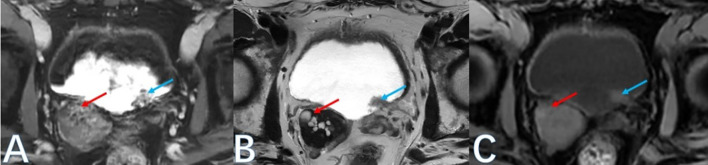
Magnetic resonance imaging (MRI) images of Case 2 demonstrating two distinct synchronous pelvic lesions. (A) Axial T1-weighted image (T1WI) showing a cauliflower-like soft-tissue lesion on the left posterior bladder wall with hypointense signal (blue arrow, ~12 × 8 mm) and a separate heterogeneous mass located in the right pelvis inseparable from the right seminal vesicle with hypointense signal (red arrow, ~3.2 × 3.1 cm). The prostate is enlarged with focal T1-hypointense areas. (B) Axial T2-weighted image (T2WI) revealing slightly hyperintense signal of the left posterior bladder wall lesion with ill-defined margins, and persistent hypointense signal of the right pelvic mass with indistinct borders. (C) Axial diffusion-weighted image (DWI, b=1000 s/mm²) showing marked hyperintensity (restricted diffusion) of the left posterior bladder wall lesion, while the right pelvic mass exhibits no definite diffusion restriction. The bladder lesion shows only mild enhancement after contrast administration, and the right pelvic mass shows heterogeneous enhancement on post-contrast sequences. No significant lymphadenopathy or pelvic fluid collection is identified. Red arrow indicates the pelvic solitary fibrous tumor located in the right pelvis; blue arrow indicates the low-grade papillary urothelial carcinoma located on the left posterior bladder wall.
